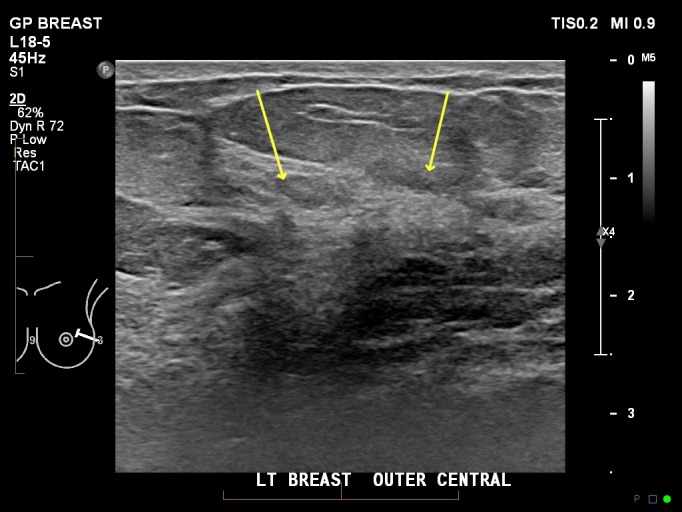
Ultrasound left outer central breast showing dense glandular tissue with minimal shadowing
